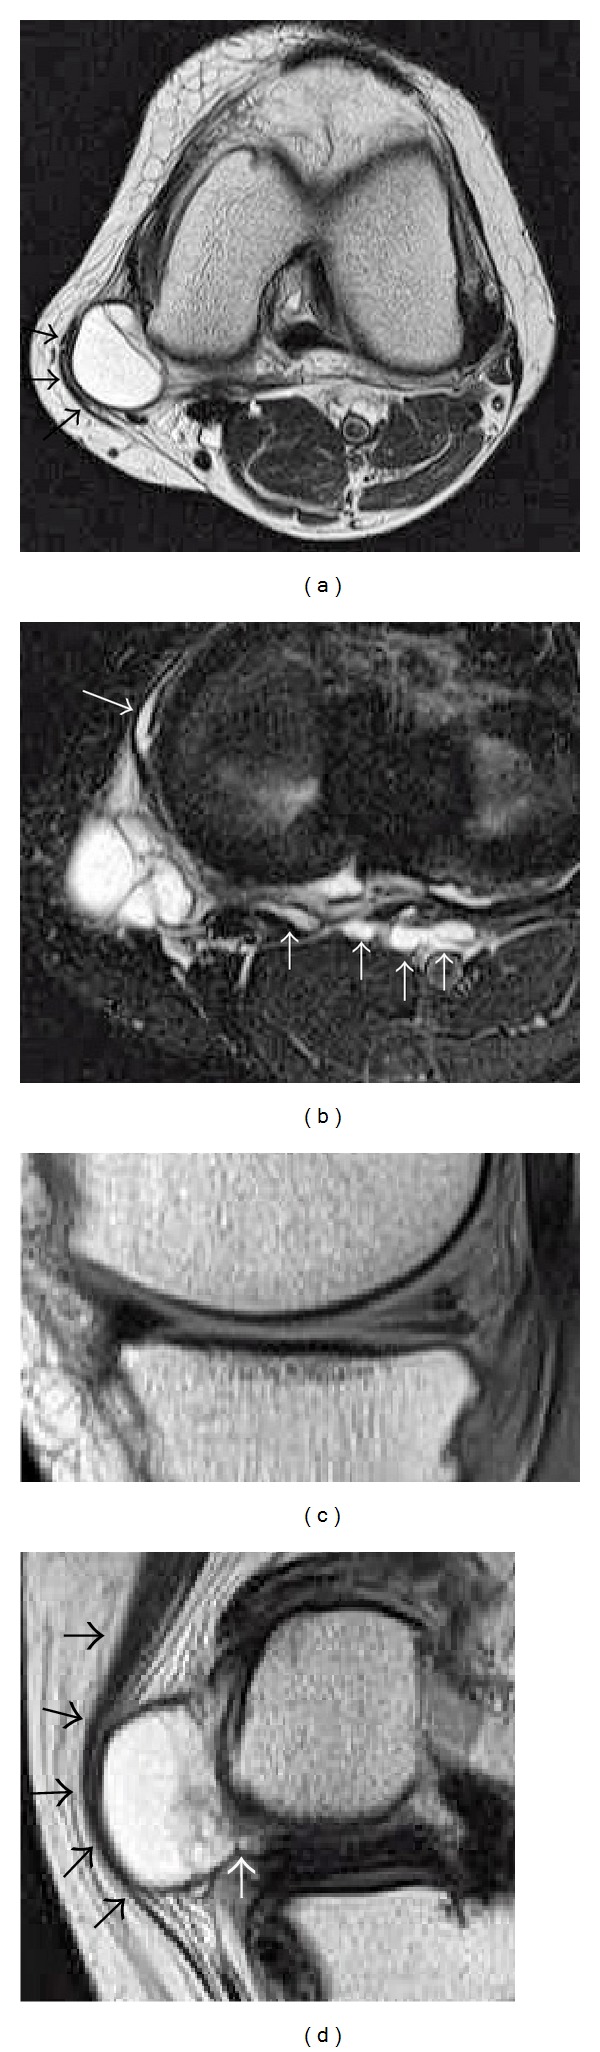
Preoperative magnetic resonance images of the left knee. (a) T2 weighted axial MRI shows a cystic mass measuring 23 mm × 20 mm detected on the posteromedial aspect of the knee. Flattened sartorius muscle (arrows) is noted just over the cyst. (b) Multiple daughter cysts expanding from the main large cyst to posterior and anterior directions were noted on STIR axial image (arrows). (c) A horizontal tear of the posterior segment of the medial meniscus was identified on sagittal proton image. (d) A communicating tract between the cyst and medial meniscus was identified (white arrow). Note that the flattened sartorius muscle is indicated by black arrows.
